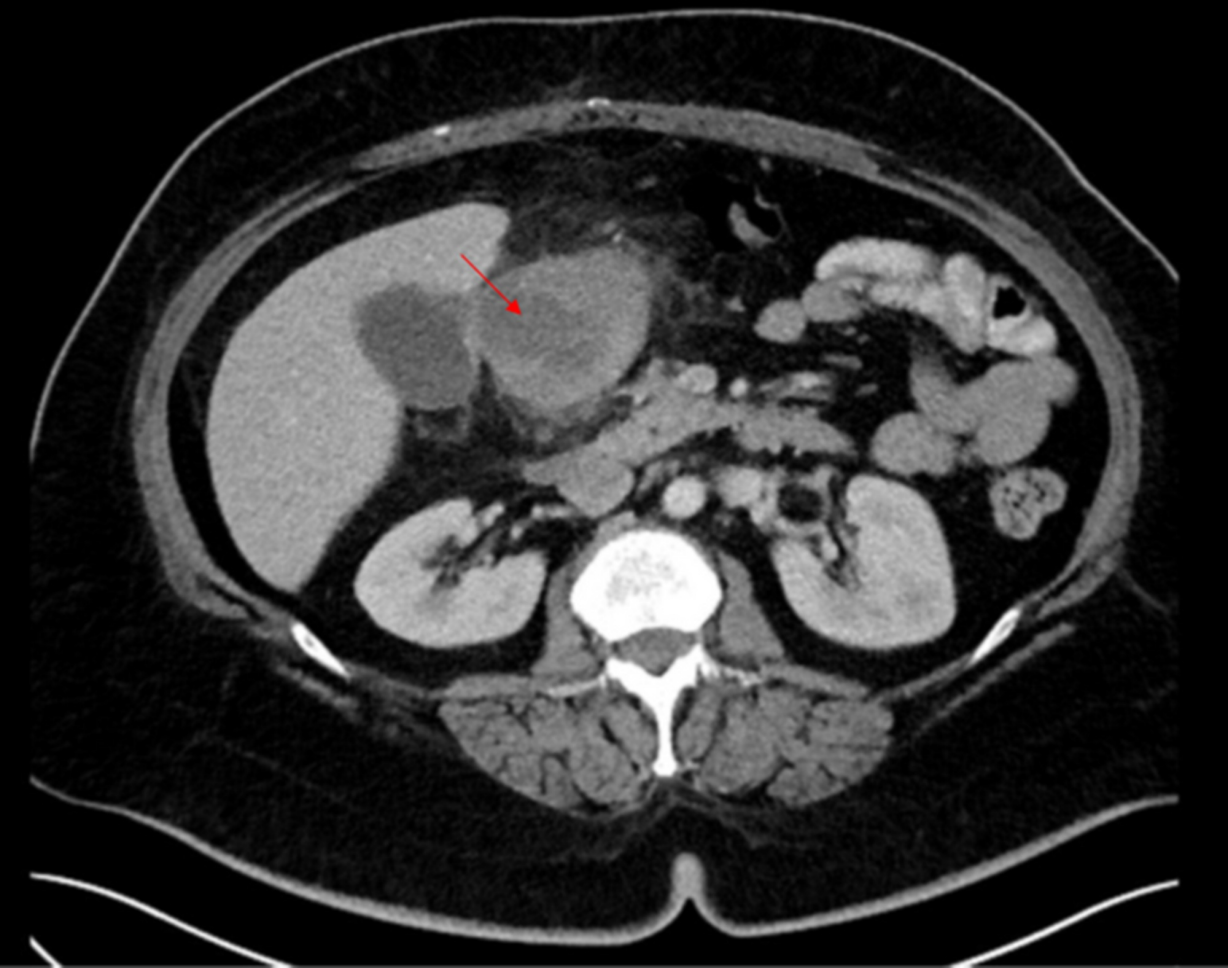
Computed tomography scan of the abdomen/pelvis showing phlegmonous gastritis
